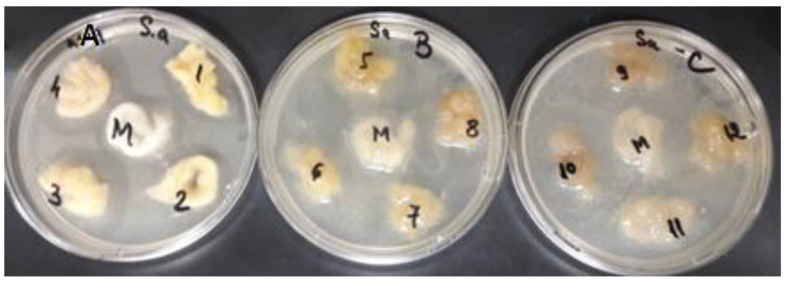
Antimicrobial activity of wool samples against *Staphylococcus aureus* ATCC 25923: A—wool samples treated with citric acid; B—UV-irradiated wool samples; C—untreated wool samples; 1—wool dyed with aqueous extract, 2—wool dyed with ultrasonic extract; 3—wool dyed with alcohol extract; 4—wool dyed with acid extract, M—control.
